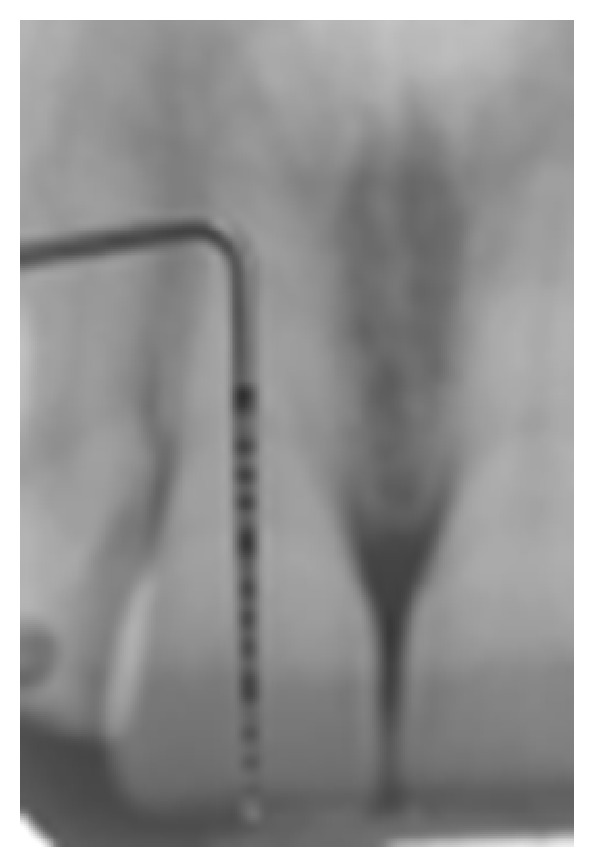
Anatomy of the tooth measured on periapical rx.
